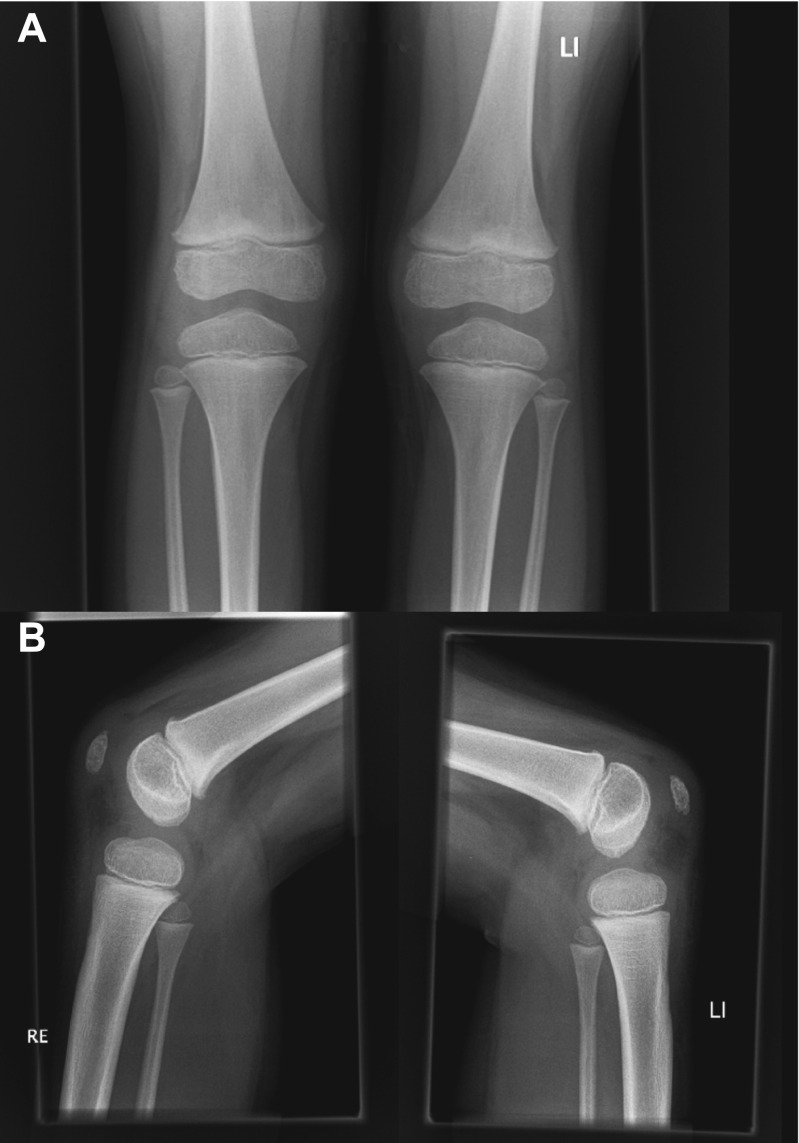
Frontal (a) and lateral (b) radiographs of both knees that were made 9.5 years earlier show no abnormalities
